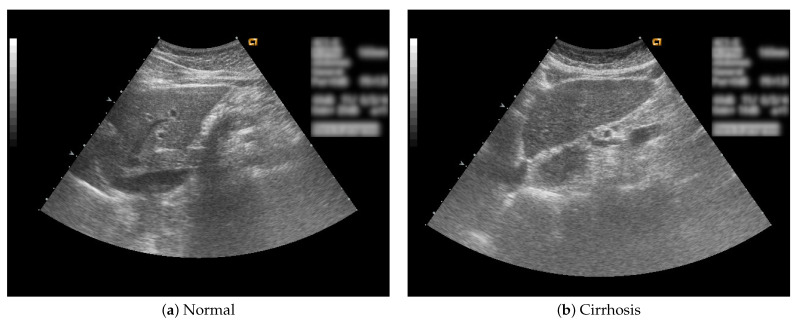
Liver ultrasound images.
